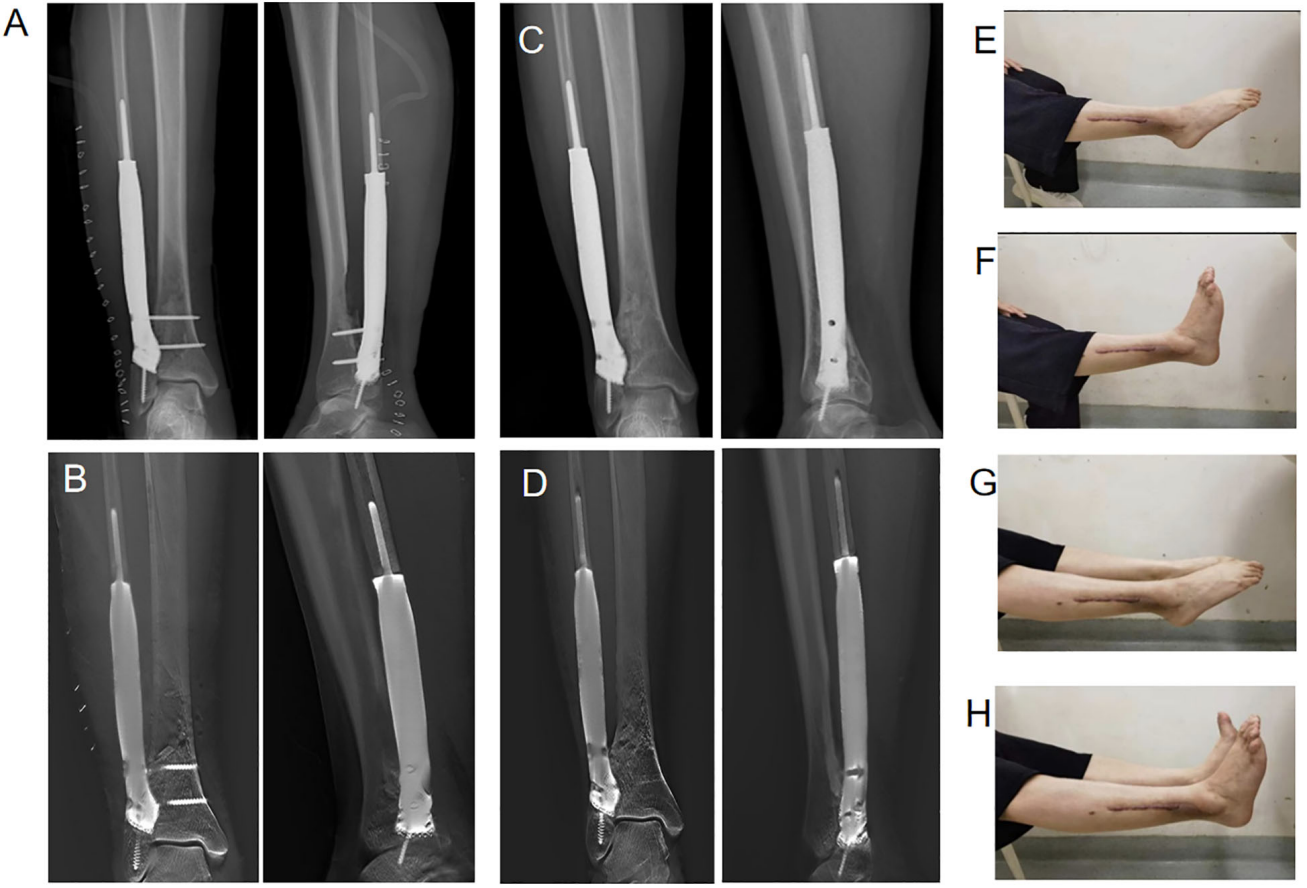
Postoperative imaging at 3 and 21 months and activity involving plantar flexion and dorsiflexion of the right ankle joint. (A) Postsurgical anteroposterior and lateral radiographs at 3 months. (B) Postsurgical anteroposterior and digital tomosynthesis images at 3 months. (C) Postsurgical anteroposterior and lateral radiographs at 21 months. (D) Following the extraction of screws from the prosthesis and tibia, anteroposterior and lateral postsurgical digital tomosynthesis revealed that the prosthesis was tightly integrated with the distal fibula, and partial bone substance was observed to grow into the pores of the prosthesis. (E, F) Activity involving plantar flexion and dorsiflexion of the right ankle joint. (G, H) Simultaneous motion of both ankles indicated that the range of motion in the ankle joint on the affected side was nearly identical to that on the healthy side.
